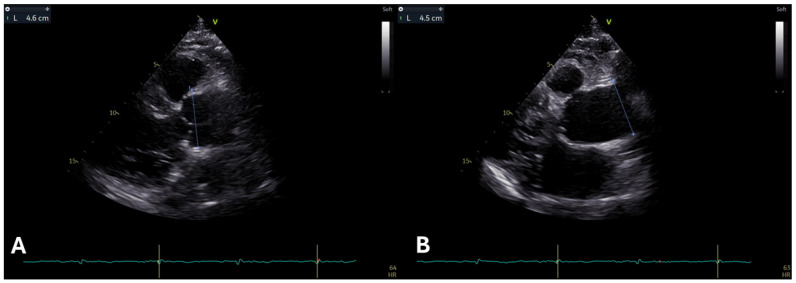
(A) A long parasternal view in 2D transthoracic echocardiography of a young adult reveals severe dilatation of the aortic root, displaying the characteristic “onion shape”. (B) A focused long parasternal view in 2D transthoracic echocardiography of the same patient demonstrates an aneurysm of the ascending aorta.
